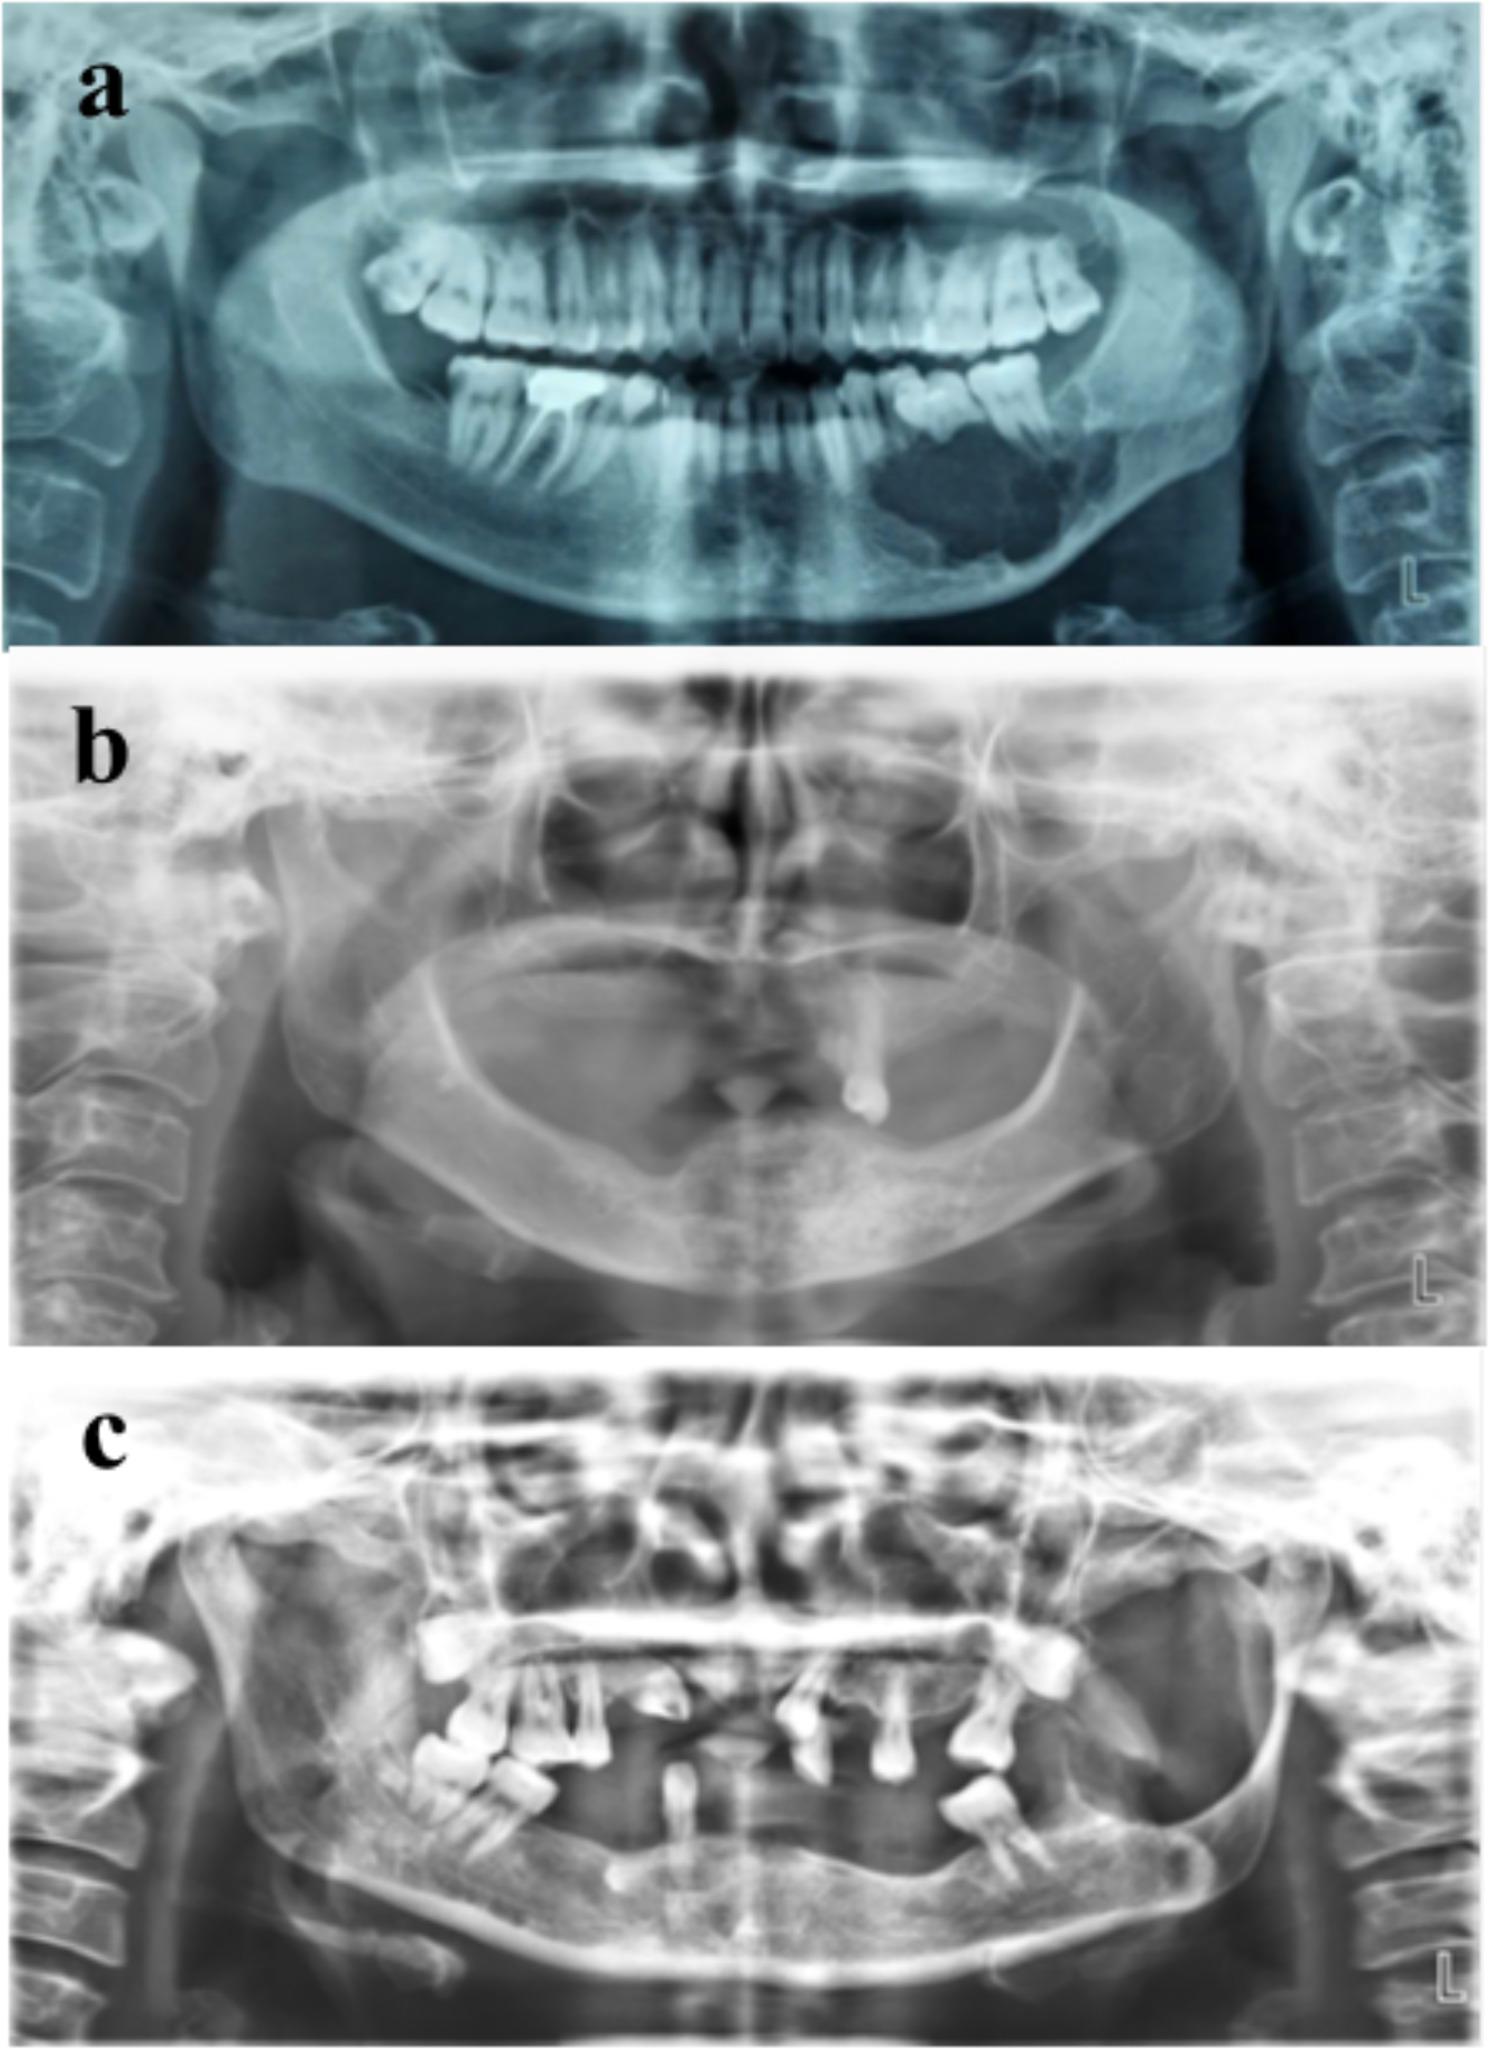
Radiographic features of mandibular ameloblastomas with different *BRAF* mutations. a Case 83: *BRAF*^V600E^ mutation, soap-bubble radiolucency with well-defined, without sclerotic margins in the left mandibular body. b Case 21: *BRAF*^D445N^ mutation, honeycomb radiolucency with ill-defined margins in the symphysis region. c Case 58: *BRAF*^V600E^ mutation, unilocular radiolucency with well-defined and sclerotic margins in the left mandibular ramus
